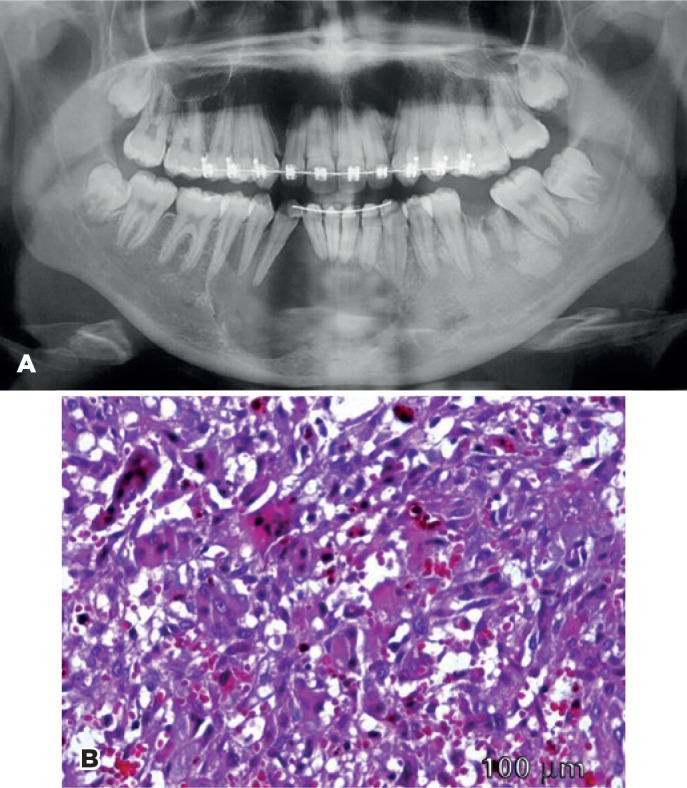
A) Radiographic imaging exhibits a radiolucent multilocular CGCG lesion
